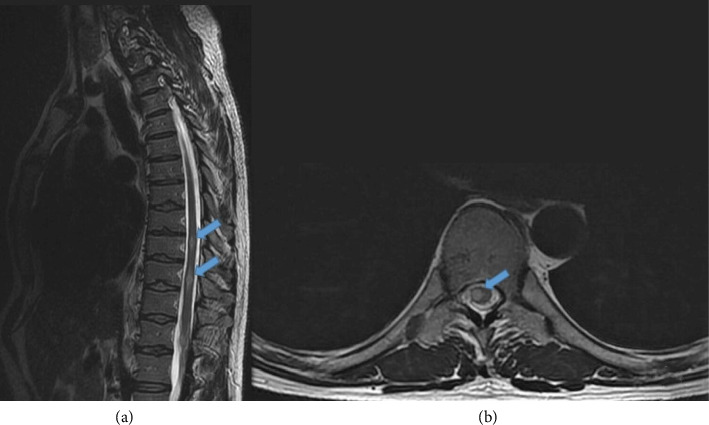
MRI of the dorsal spine with T2 (a, b) sequences. High-signal T2 plaques of variable size between 5 and 12 mm are observed, located in patchy form along the entire dorsal spine, compatible with myelitis in relation to a paradoxical reaction to tuberculosis treatment.
